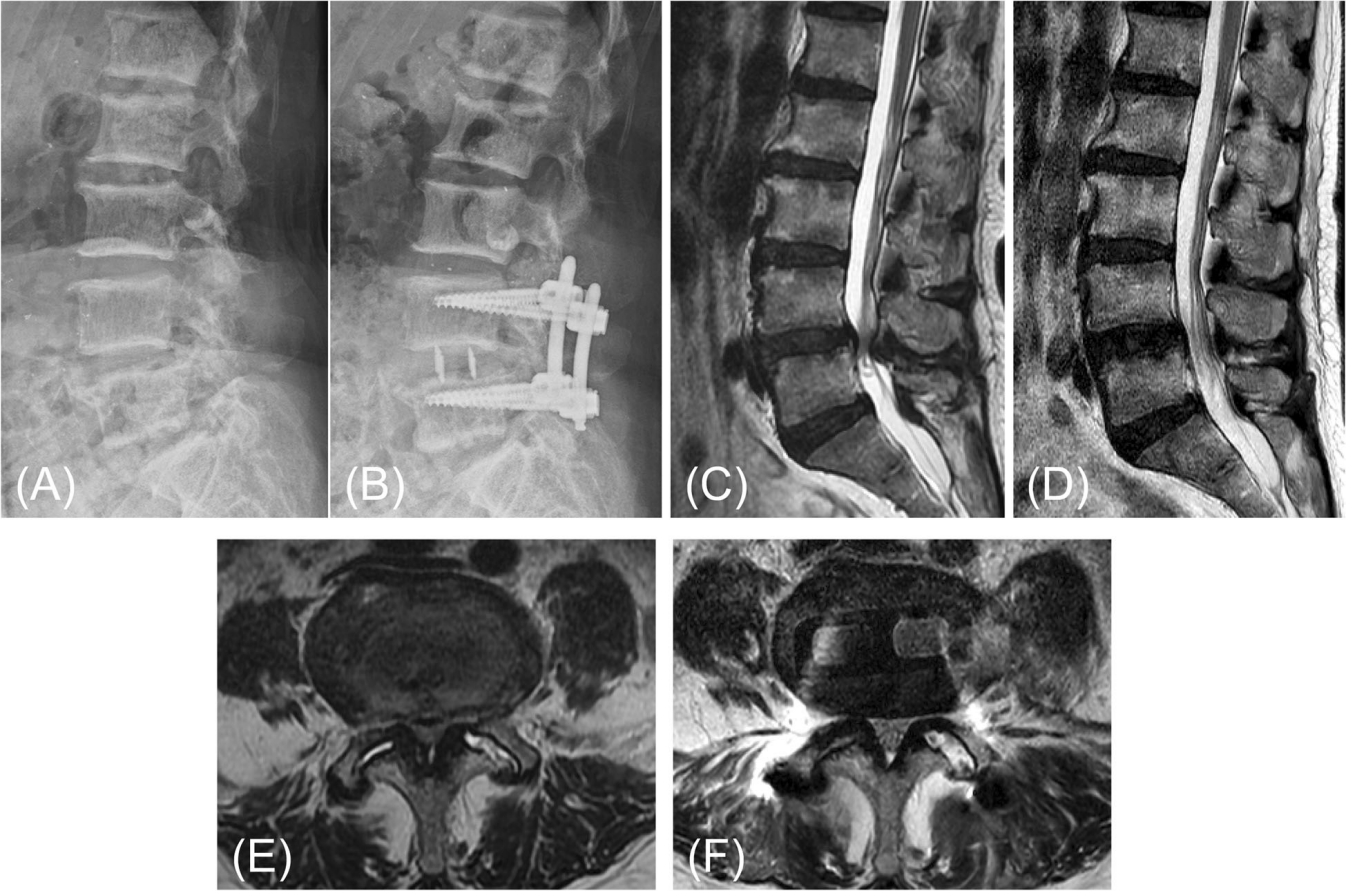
L4 degenerative spondylolisthesis with lumbar canal stenosis in a 65-year-old female (patient No. 15 in Table 1). a Lateral radiograph before surgery. b Lateral radiograph 2 months after surgery (XLIF plus SP-PPS in lateral decubitus position). Sagittal and axial magnetic resonance imaging before surgery (c, e) and 2 weeks after surgery (d, f). An increase in cross-sectional spinal canal area (CSA) (from 58.1 mm^2^ to 66.8 mm^2^) and diameter (from 4.2 mm to 9.1 mm) were observed after surgery, but the change was small at 2 weeks after surgery. However, the patient’s preoperative symptoms have improved, and she is progressing satisfactorily after the surgery
